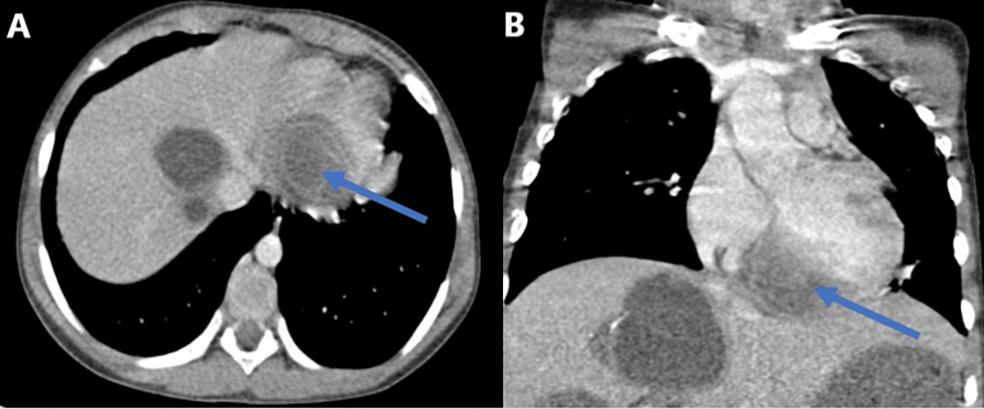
Axial (A) and coronal (B) computed tomography showing the cystic lesion in the interventricular septum (arrows).
